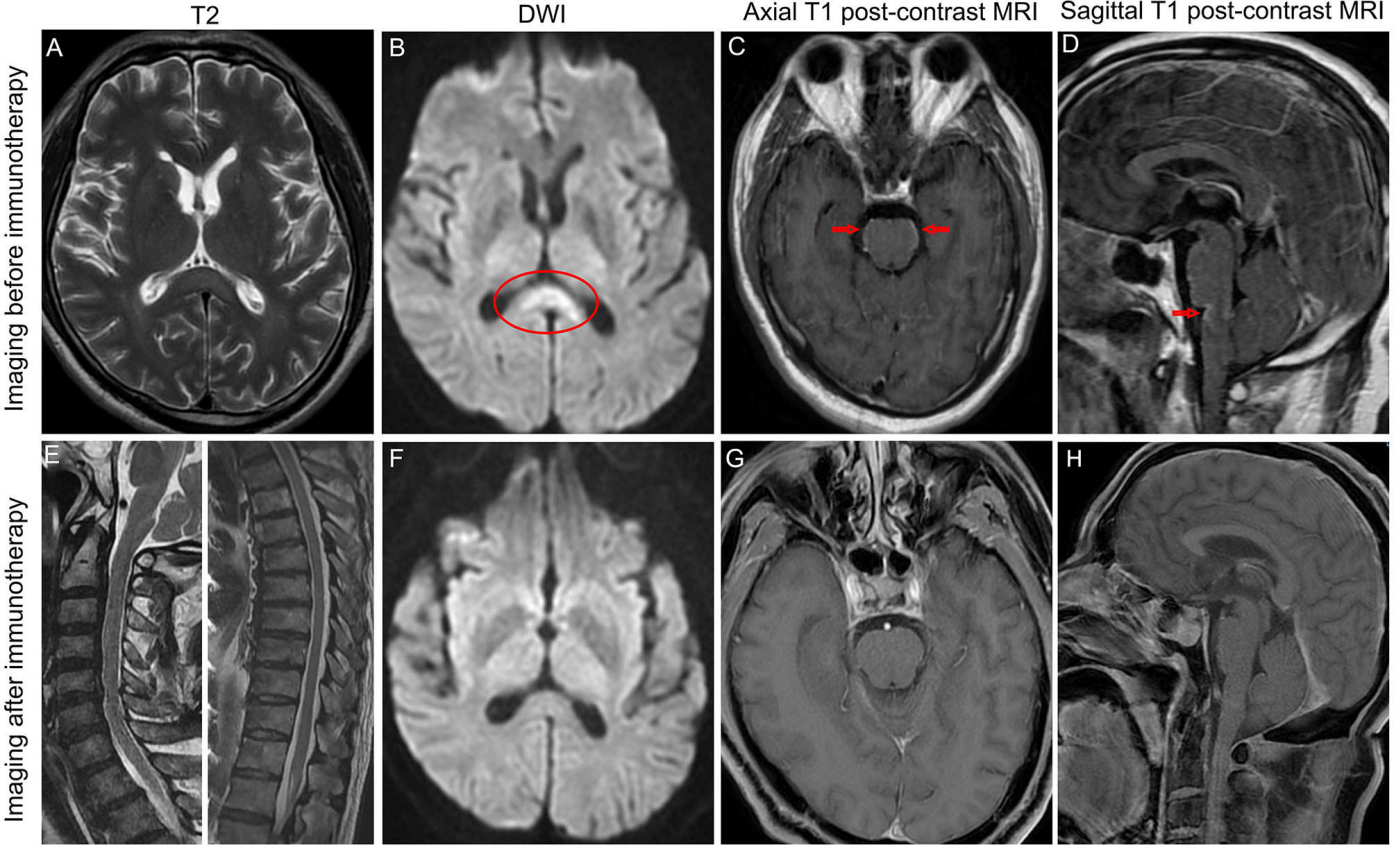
Brain MRI revealed hyperintense of the corpus callosum on T2 sequence MRI (A) and diffusion-weighted MRI (B) before immunotherapy. MRI postcontrast axial T1-weighted scans (C) and sagittal T1-weighted scans (D) showed leptomeningeal enhancement of the pons and medulla before immunotherapy. Sagittal T2 sequence MRI showed no hyperintensities throughout the cervical spinal cord and thoracic spinal cord (E). The high signal intensity of the corpus callosum on diffusion-weighted MRI disappeared after immunotherapy (F). MRI postcontrast axial T1-weighted scans and sagittal T1-weighted scans showed that leptomeningeal enhancement of the pons and medulla completely disappeared after immunotherapy (G, H). Arrows indicate leptomeningeal enhancement. Oval shape indicates the lesion in the corpus callosum.
